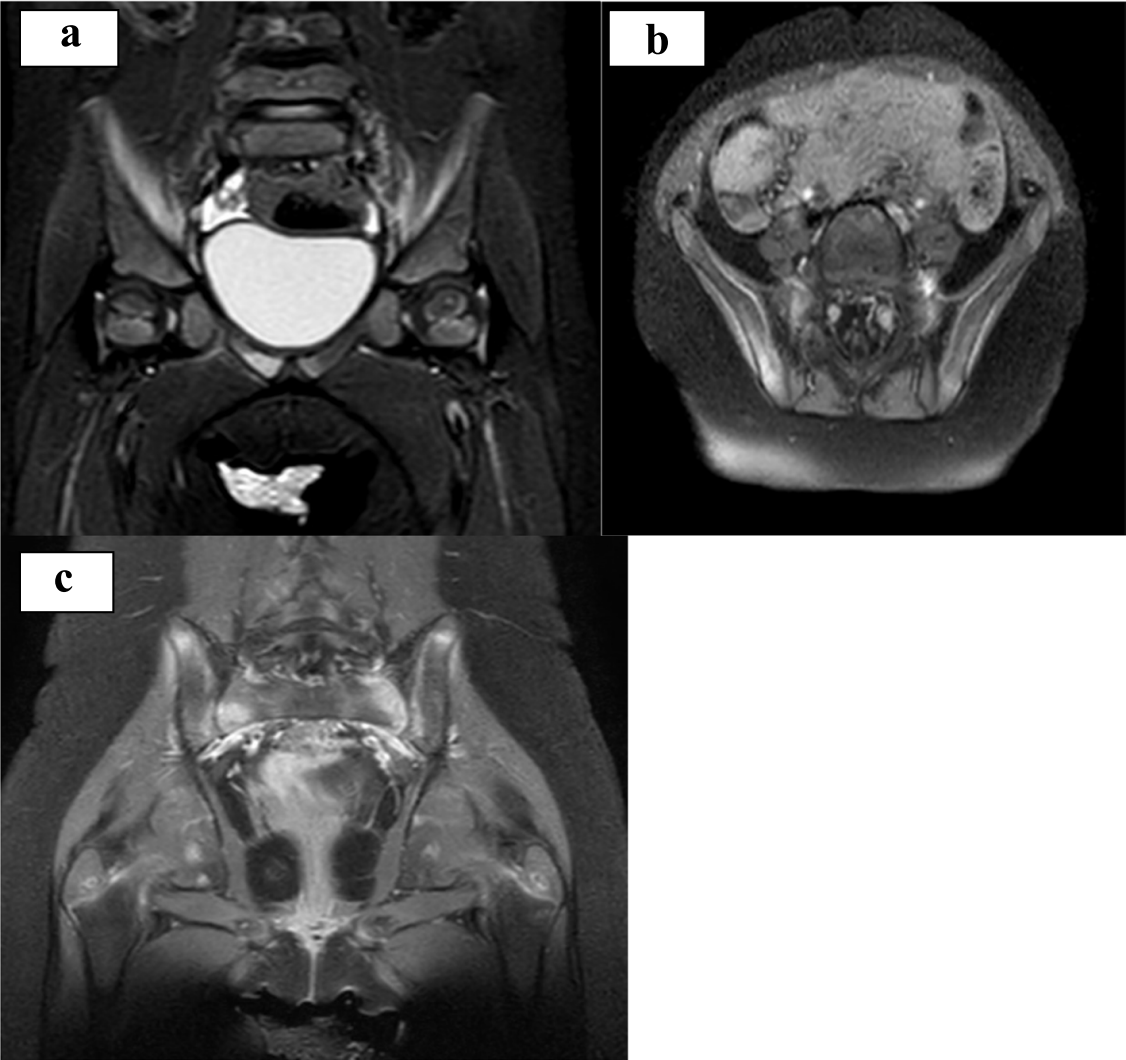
Pelvis MRI at disease onset. a The T2w sequence documents some areas of altered signal referable to spongious edema, some of them with symmetrical distribution, blurred, involving the limiting joints of the sacro-iliac synchondroses and more cranially the iliac crests, others with asymmetrical distribution, in the presence of conspicuous edematous alteration of the pre-symphyseal side of the right ileo- and ischio-pubic branches that on the opposite side seems only veiledly hinted at. b, c Changes are accompanied by discrete contrastographic impregnation in the CE-FS T1w sequence, particularly that located at the pubic site on the right, where concomitant periosteal reaction is seen, with traces of initial edema of the adjacent adductor muscle bundles
